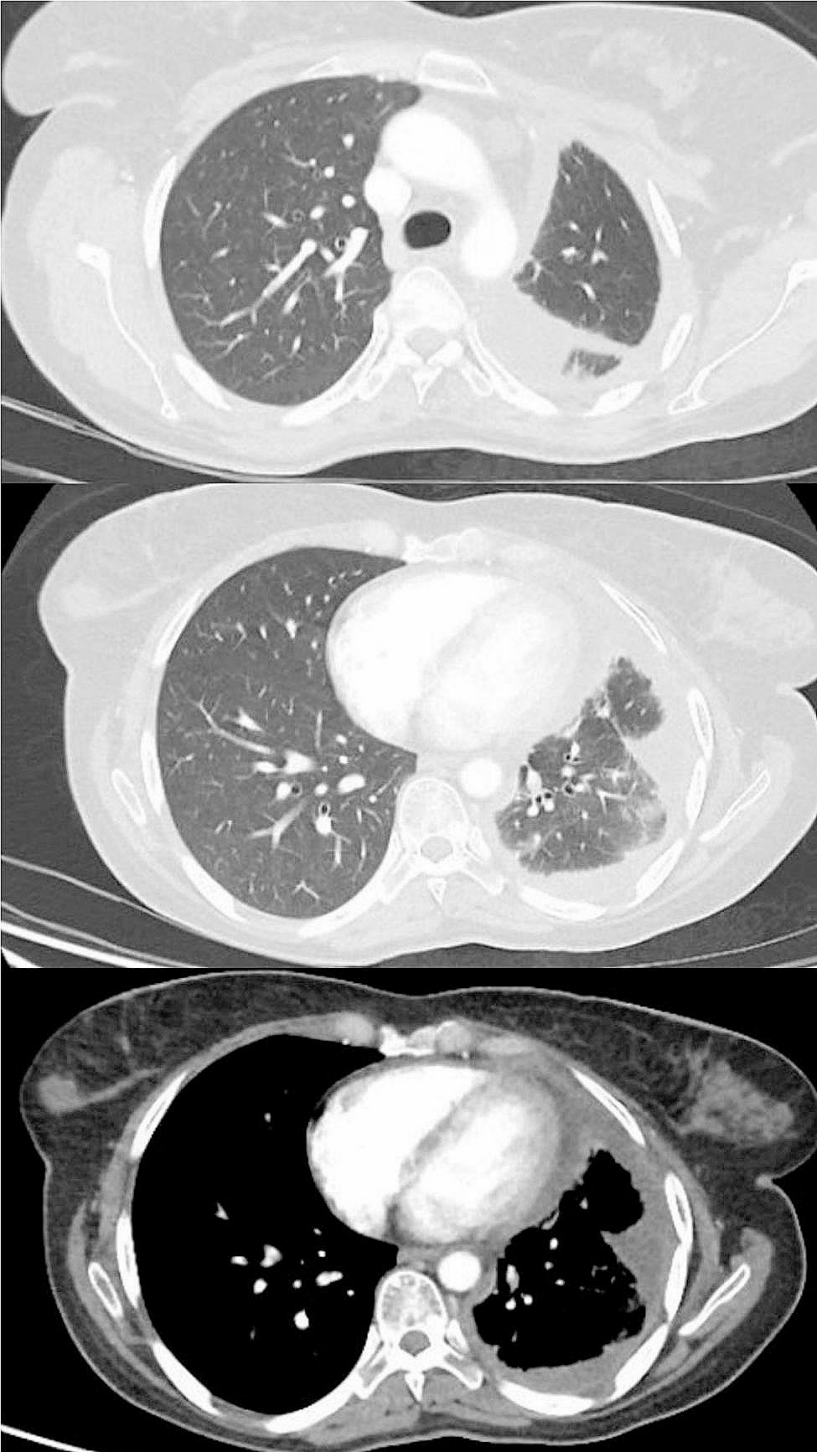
Computed tomography scan of lungs demonstrating left-sided pleural effusion, and minimal pleural thickening, without accompanying parenchymal abnormalities
